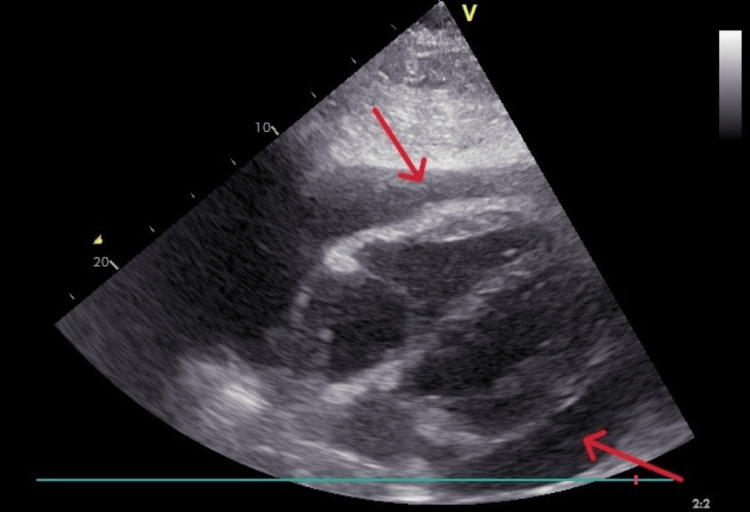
Transthoracic echocardiography image shows a significant volume pericardial fluid (red arrows).
